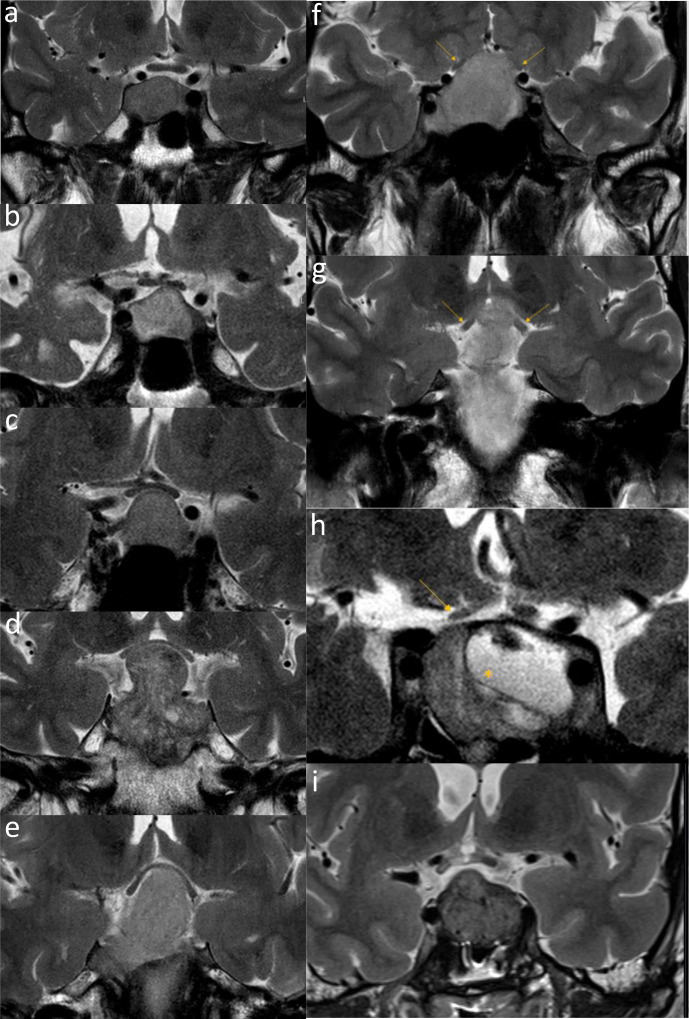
Coronal T2 weighted images showing different grades of compression on the optic system and hyperintensity of the optic nerve. Examples of the radiological scoring used in the present study are shown. Notably, all panels show radiological examination of different patients performed at different points in time with different MRI settings. (A–E) The five Fujimoto classification grades are: 0, tumor has no contact with optic chiasm; 1, tumor has contact with optic chiasm without deformity of the upper surface of optic chiasm; 2, tumor compresses the optic chiasm and produced the deformity of the upper surface and visible supra-chiasmal cistern; 3, tumor compresses the optic chiasm with invisible supra-chiasmal cistern; 4, tumor compresses the optic chiasm with cerebral deformity. (F) Compression of the prechiasmatic optic nerve: the adenoma has contact with the left optic nerve without any deformation (grade 1) and compresses and deforms the right optic nerve (grade 2). (G) Contact with the optic tract: the adenoma extends to the left optic tract (grade 1) while there is no contact with the right optic tract (grade 0). (H) Atrophy and increased signal intensity of the right optic nerve (arrow) in a patient with a mixed solid-cystic macroadenoma (asterisk) reaching up to the chiasm (I) Increased signal intensity without atrophy in the right optic tract.
